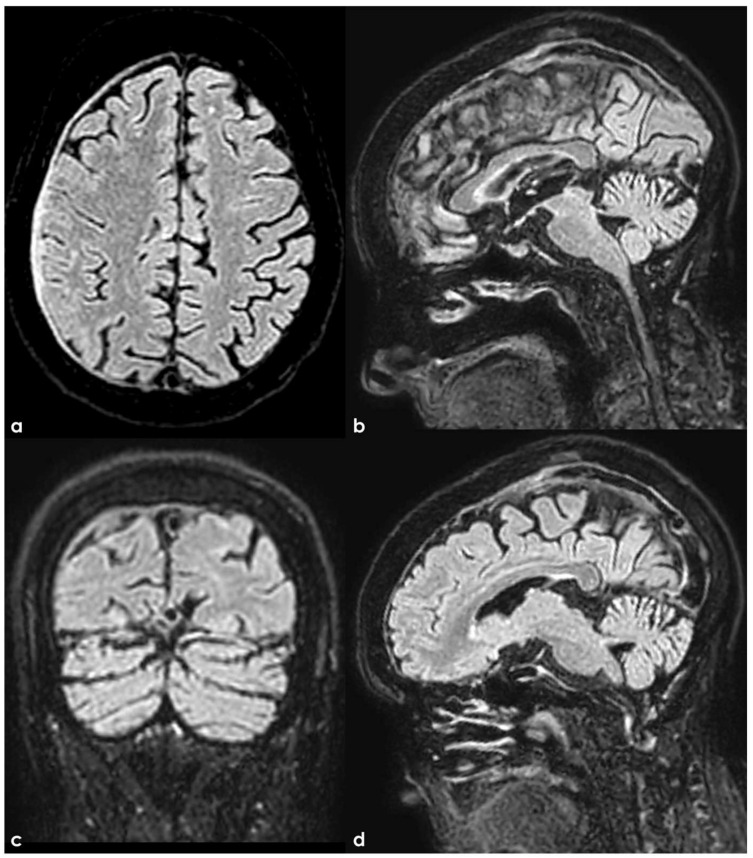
An example of SSS septations and associated AG. Here, 3D-FLAIR images are reconstructed in MPR in the axial (panel (a)), sagittal (panel (b,d)), and coronal (panel (c)) planes. The section of SSS in panels (a,c) seems to have a double lumen, and in panels (b,d), a long tortuous hyperintense septation within the SSS is recognizable starting near to a discrete rounded AG, with hyperintense boundaries and filling content with the same signal as CSF.
